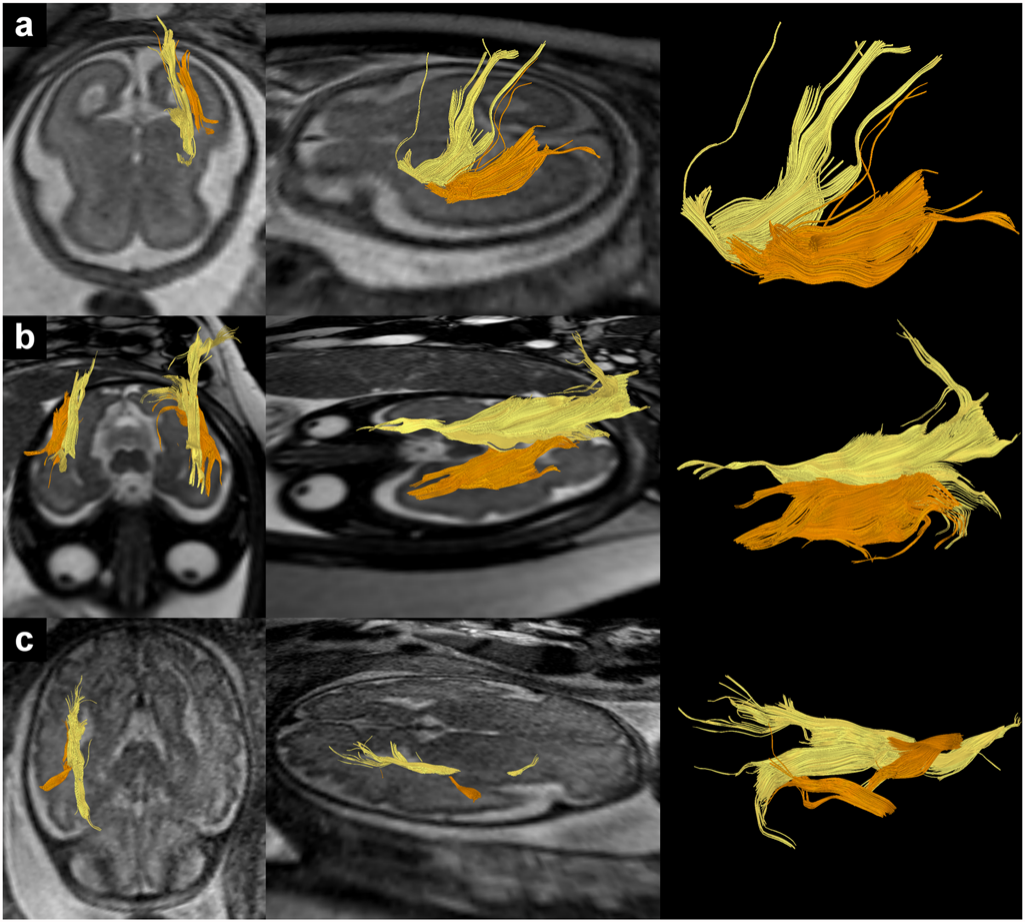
In utero tractography of the ILF (orange) in subjects 4 (a), 19 (b) and 24 (c) (23, 33 and 34 GW respectively) and its relation to the IFOF (yellow). Fiber tracts are projected on axial T2-weighted images.
